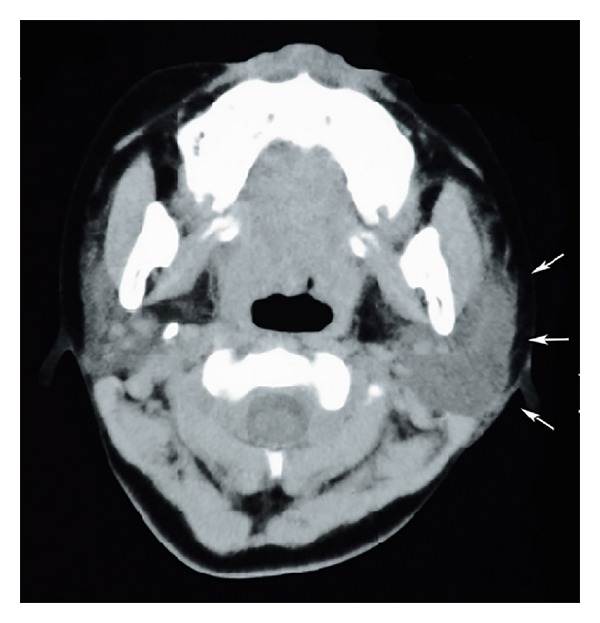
A computed tomography image of the neck reveals the swelling of the left parotid gland (arrows).
